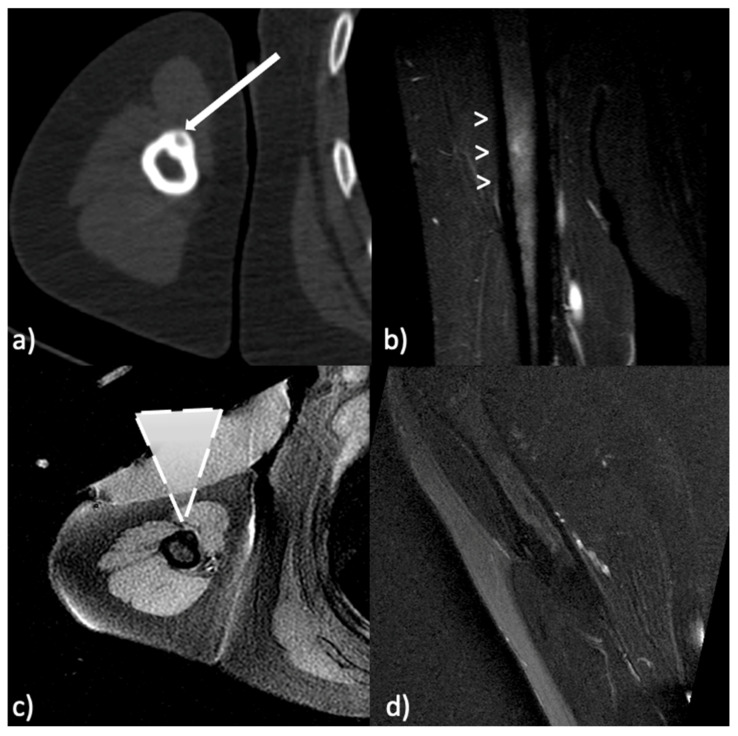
Osteoid osteoma of the omerus. (a) Pretreatment computed tomography (CT) shows the nidus (arrow) of the osteoid osteoma. (b) Pretreatment T2-weighted fast spin-echo magnetic resonance (MR) with fat-saturation shows bone marrow edema around the nidus (arrowheads). (c) MR image during treatment with the ultrasound (US) transducer in contact with the skin (dashed triangle represents the US beam focused on the target). (d) 1-year follow-up T2-weighted fast spin-echo MR with fat-saturation shows resolution of the bone edema.
